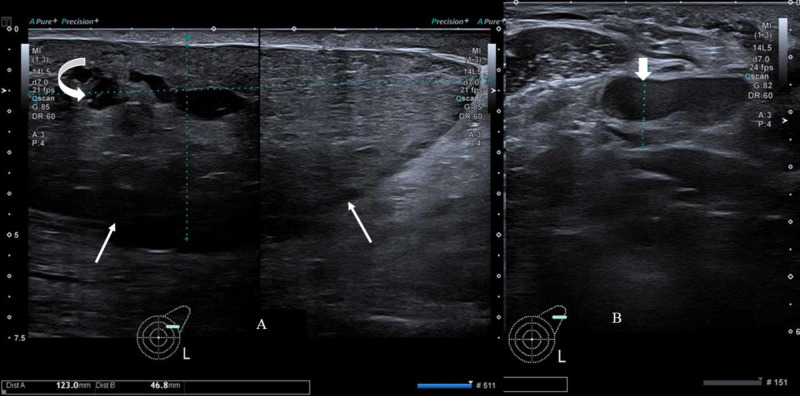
Gray scale ultrasound image of left breast and axilla (A) Image shows a solid, circumscribed mass (thin white arrows) predominantly in upper outer quadrant of left breast with few cystic spaces (curved arrow). (B) Image shows abnormal left axillary lymph node with asymmetric cortical thickening (short thick arrow) and displaced hilum.
